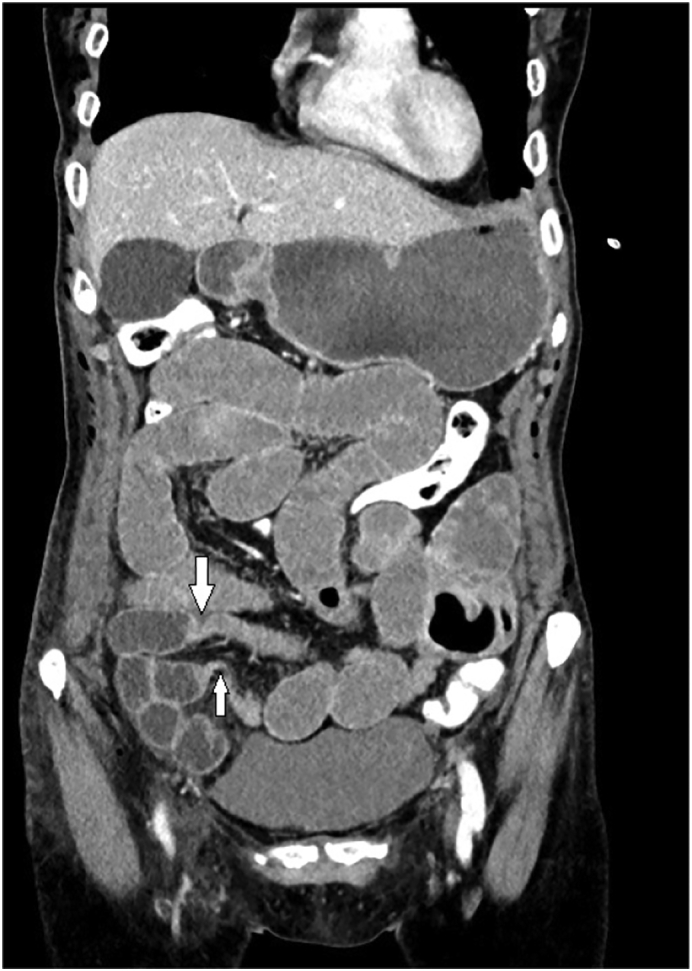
CT Abdomen with evidence of a small bowel obstruction at two contiguous points (white arrows) by means of closed loop obstruction. See also dilated small bowel loops proximal to closed loop site.
